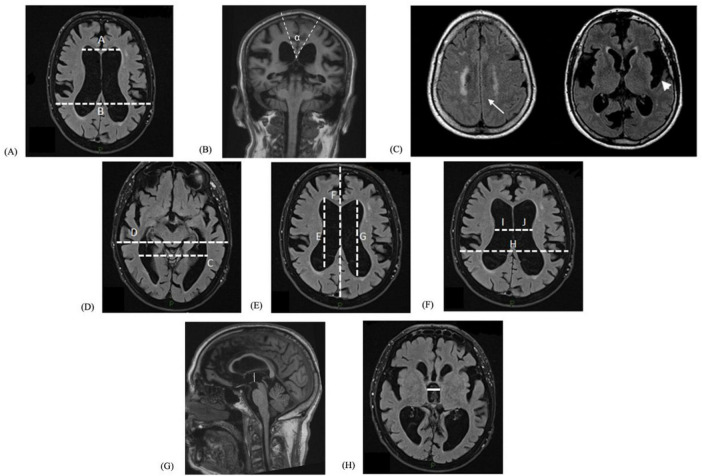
Linear radiological indexes. (A) Evans’ Index: maximal width of the frontal horns (A) and the largest internal diameter of the cranium at the same slice (B). (B) Callosal angle: the angle is calculated at the level of posterior commissure (α). (C) Disproportionately Enlarged Subarachnoid Space Hydrocephalus: narrowing of the subarachnoid spaces near the vertex (arrow) and widening of the Sylvian fissures (arrowhead). The hyperintense periventricular white matter abnormalities in both axial FLAIR sequences are referred to slight chronic microvascular damage. (D) Magnetic Resonance Hydrocephalic Index: the largest left-to-right width of the collateral trigones of the lateral ventricles (C) and maximum inner skull diameter (D). (E) Anteroposterior diameter of the Lateral Ventricle Index, evaluated for each side: anteroposterior diameter of each lateral ventricle (E and G, right and left, respectively) and the maximal width of the anteroposterior inner diameter of the skull (along the cerebral falx) (F). (F) Modified Cella Media Index, assessed for both sides: the largest internal diameter of the cranium (H) and the maximum cella media width of each lateral ventricle (I and J, right and left, respectively). (G) Height of the third ventricle. (H) Width of the third ventricle.
